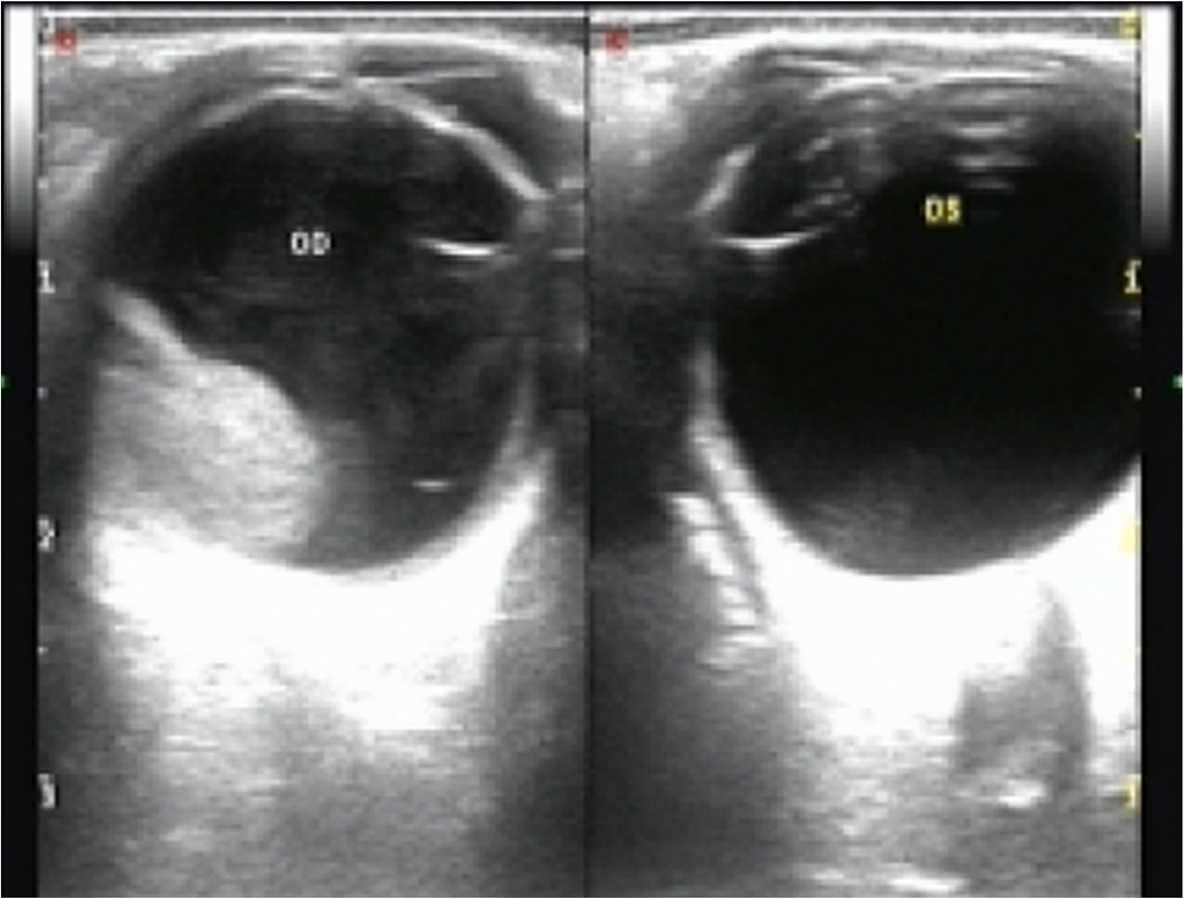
Color ultrasound examination of the eyeson presentation demonstrating dense vitreous opacities and avascular homogeneous hyperechoic mass in the right eye (OD), normal in the left (OS)
